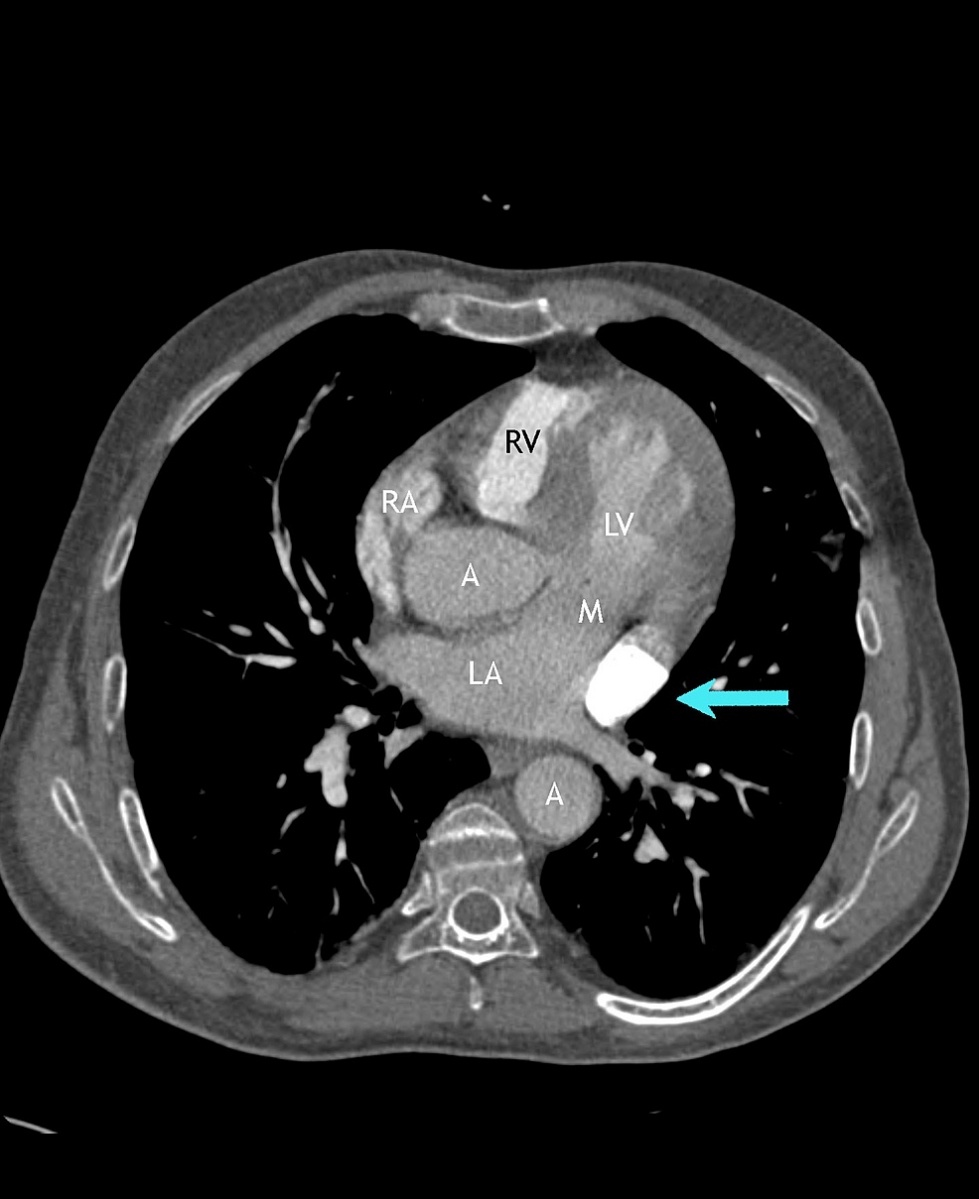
Axial CT angiogram following contrast injection in the right subclavian vein showing the isolated PLSVC (arrow) without evidence of any right-sided SVC draining into the normal position at the level of the right atrium LA: left atrium, LV: left ventricle, RA: right atrium, RV: right ventricle, M: mitral valve, A: aorta
